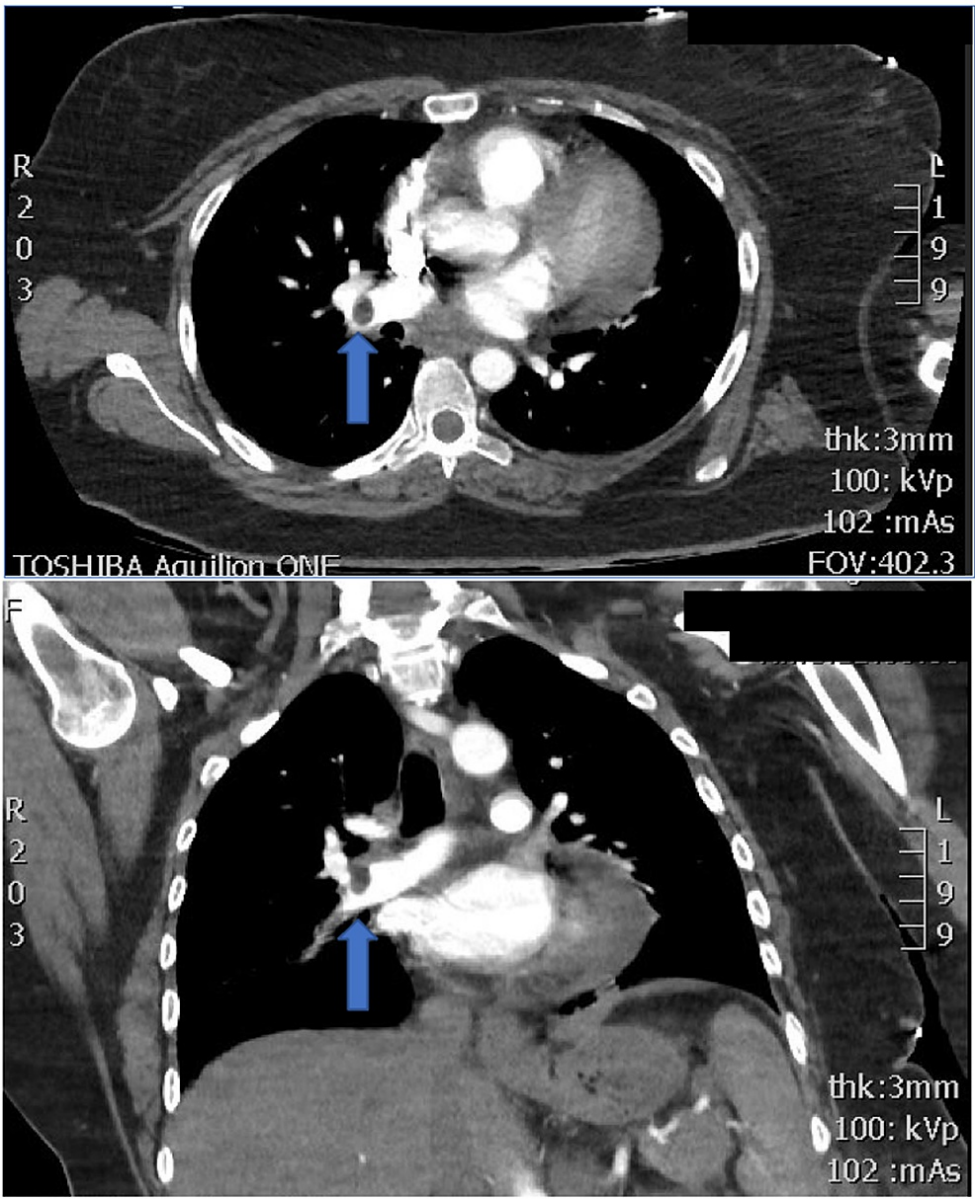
CT pulmonary angiogram - right lower lobe pulmonary embolus.
